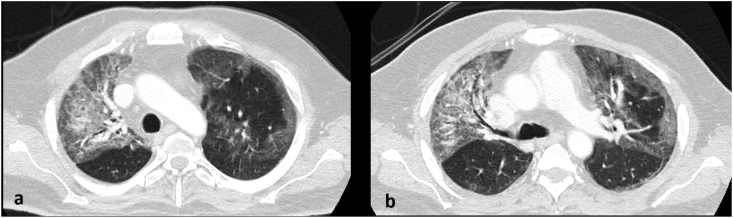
Chest CT (a and b) during active COVID-19 infection in a 58-year-old patient (#21 in Table 4) showing bilateral lung abnormalities (CO-RADS 6) suggestive of interstitial pneumoniae.
